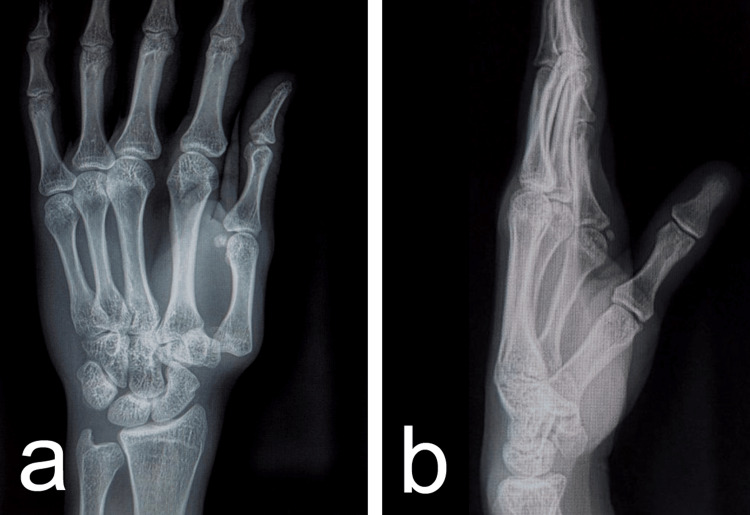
Diagnostic anteroposterior (a) and lateral (b) radiographs showing dorsal dislocation of the first carpometacarpal joint.
